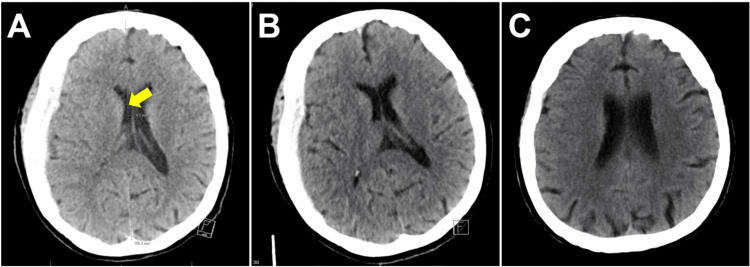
(A) Axial view of computed tomography scan of the brain provides evidence of a 4-millimeter midline shift (yellow arrow). In (B) and (C), repeat scans show interval improvement and resolution, respectively, without the need for neurosurgical intervention.
